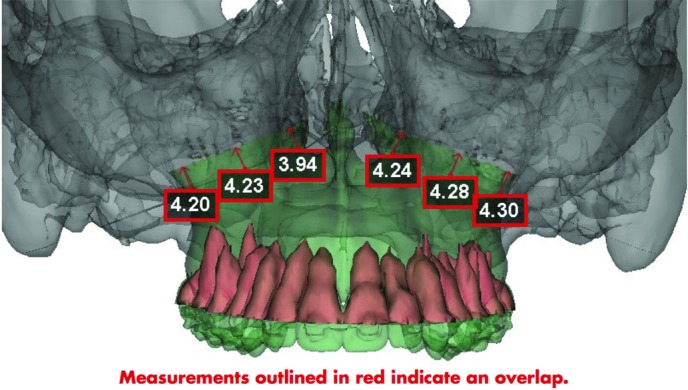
Computed tomographic scan with 3-dimensional reconstruction view of planned Le Fort I impaction. The numbers indicate movement of the maxilla in the superior vector (millimeters).
